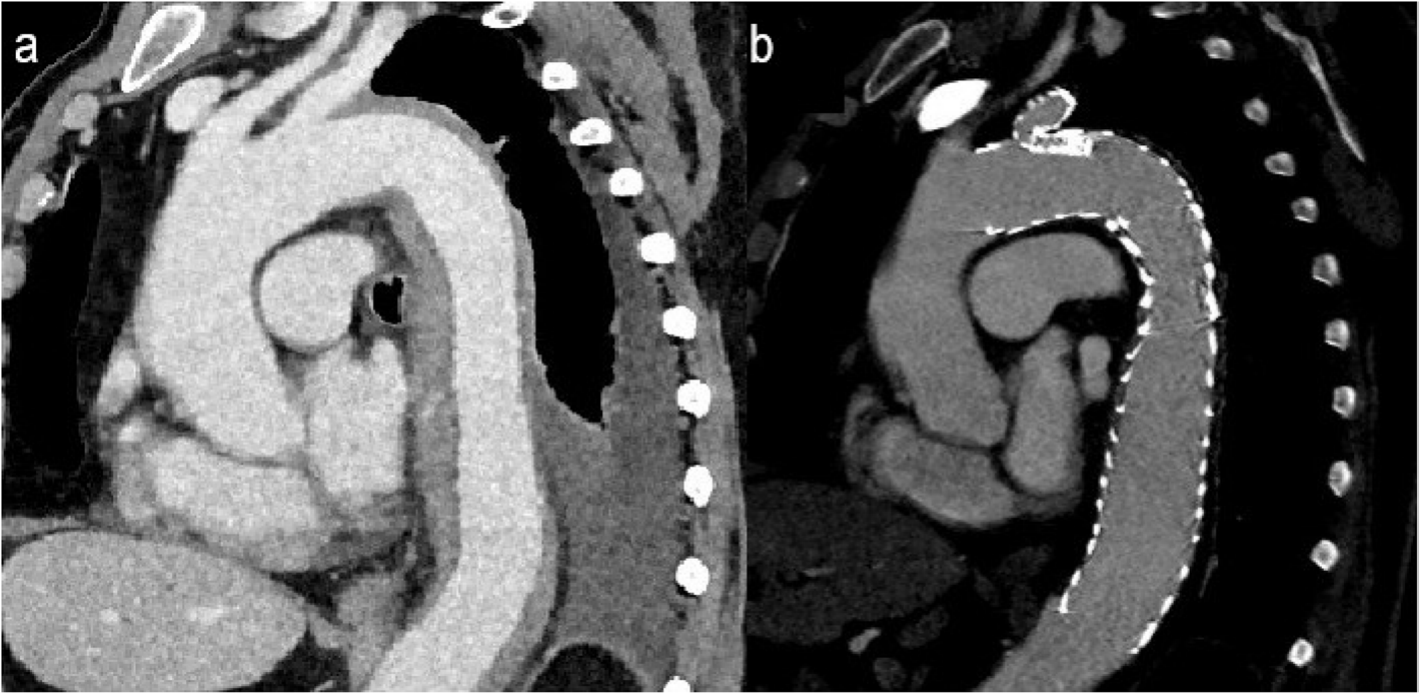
Saggital CTA reconstructed images of a patient with an intramural hematoma before (a) and after (b) treatment with the single-branched stent graft
